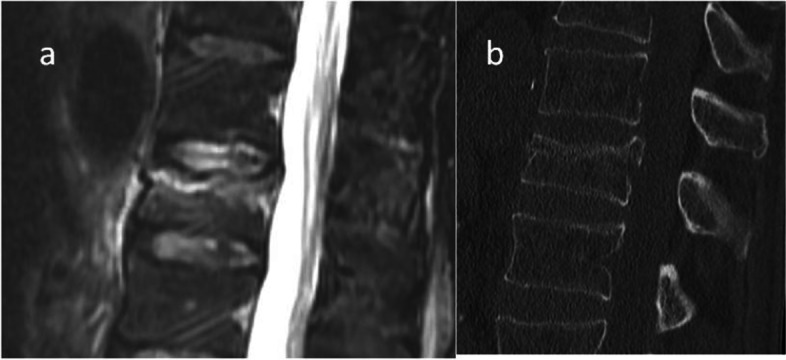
Depicted is a case with an incomplete burst fracture type OF 3. There was a perfect agreement for both MRI only (a) and in combination with MRI and CT (b)
